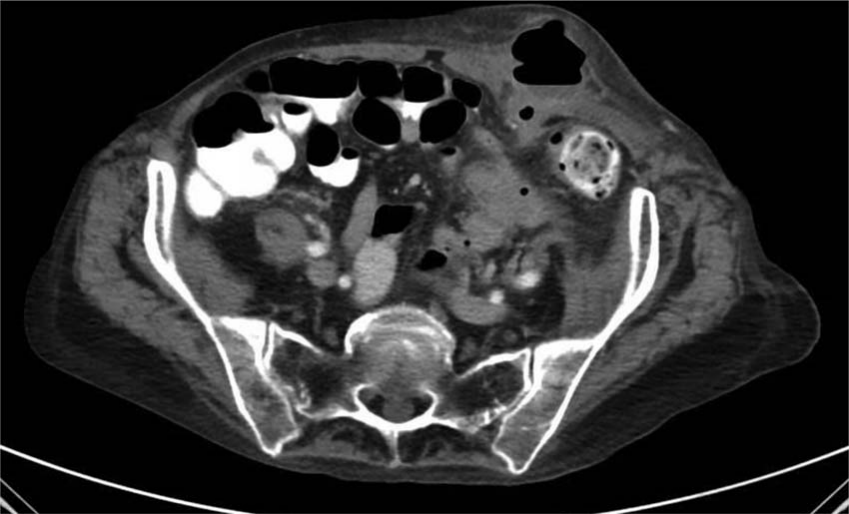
CT from readmission with anterior abdominal wall abscess and left pelvic abscess.
